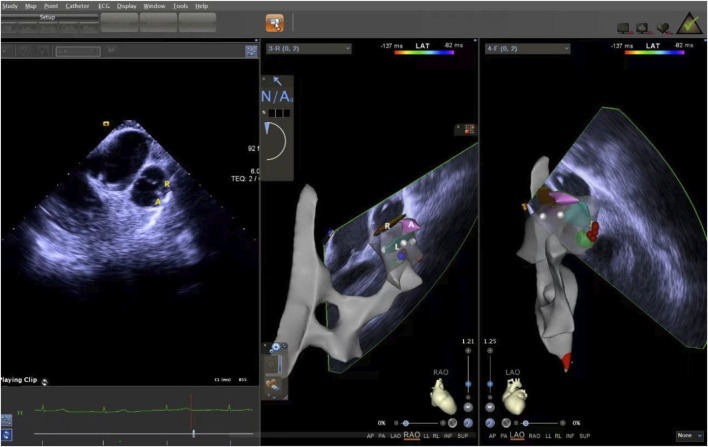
ICE was used to observe the short-axis section of the pulmonary sinus. ICE, Intracardiac echocardiography.
